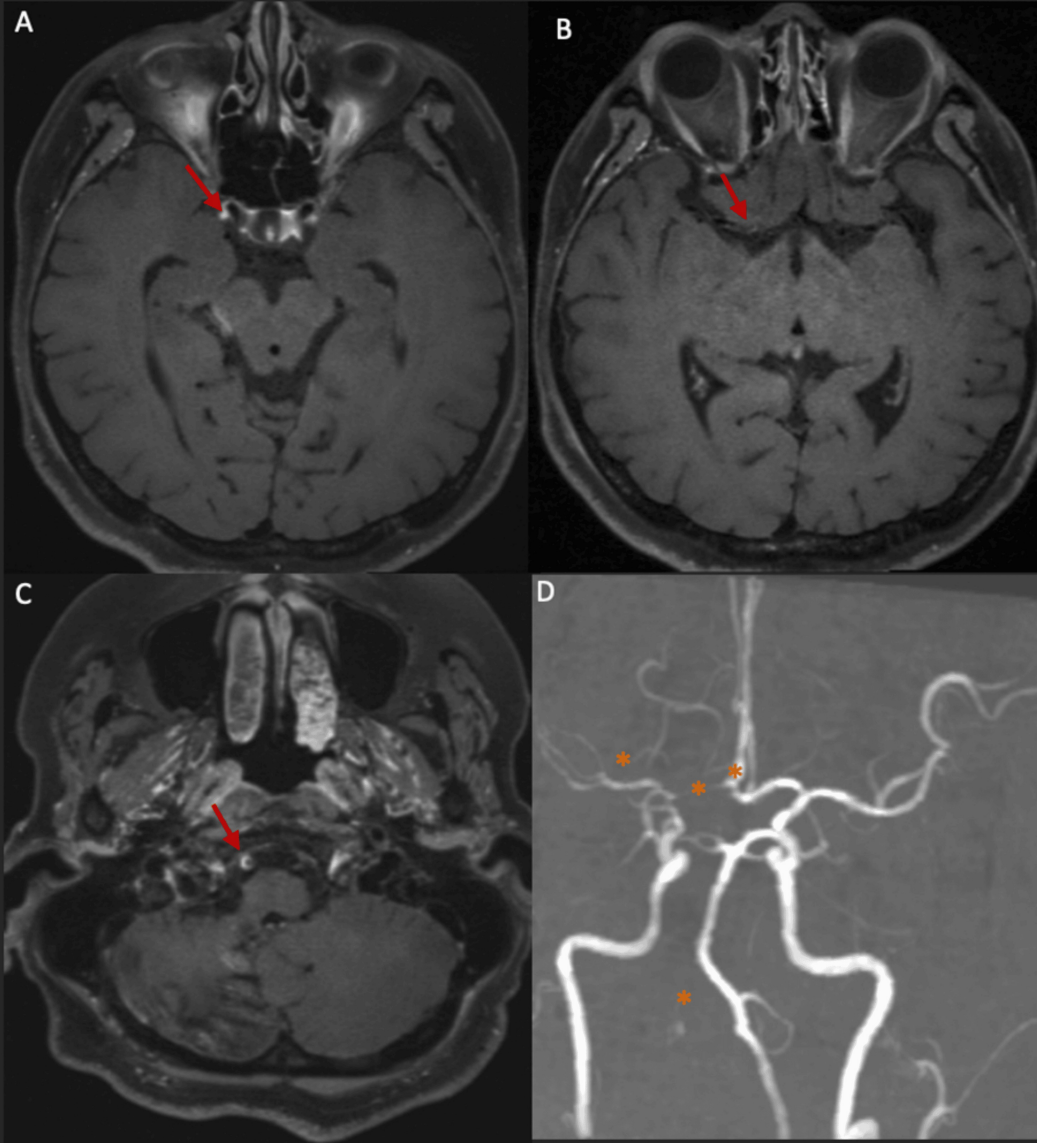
Follow-up contrast-enhanced brain MRI with high-resolution vessel wall imaging ((A–D) (A–B) Post-contrast T1-weighted black-blood high-resolution vessel wall images demonstrate interval reduction in concentric wall thickening and enhancement compared with the prior study (red arrows). (C–D) Persistent luminal narrowing with residual enhancement is noted in the right vertebral artery (V4 segment) and the right middle cerebral artery (M1 segment), without new vascular territories involved (orange asterisks).
